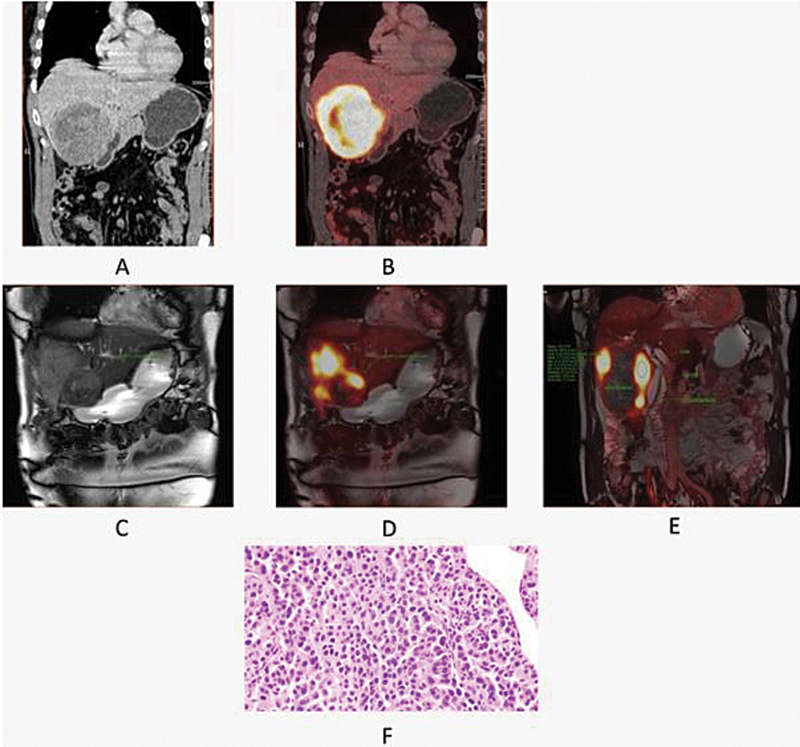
Case of hepatocellular carcinoma abutting adjacent structures: This figure shows the advantage of PET/MRI in revealing additional information about the hepatic mass as compared with PET/CT. (
